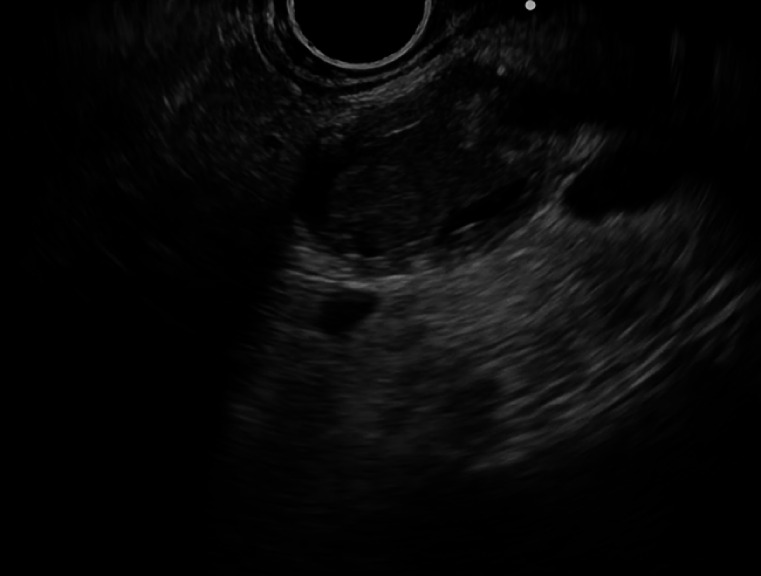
Endoscopic ultrasound image showing an intraductal lesion, with thickening of the wall in the main pancreatic duct.
